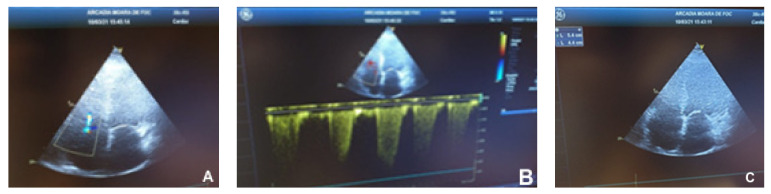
Dilated cardiomyopathy echocardiogram. (A)—Mild tricuspid regurgitation allowing the measurement of (B)—right ventricular–right atrial gradient and the estimation of systolic pulmonary artery pressure. (C)—diminished tricuspid annular plane systolic excursion.
